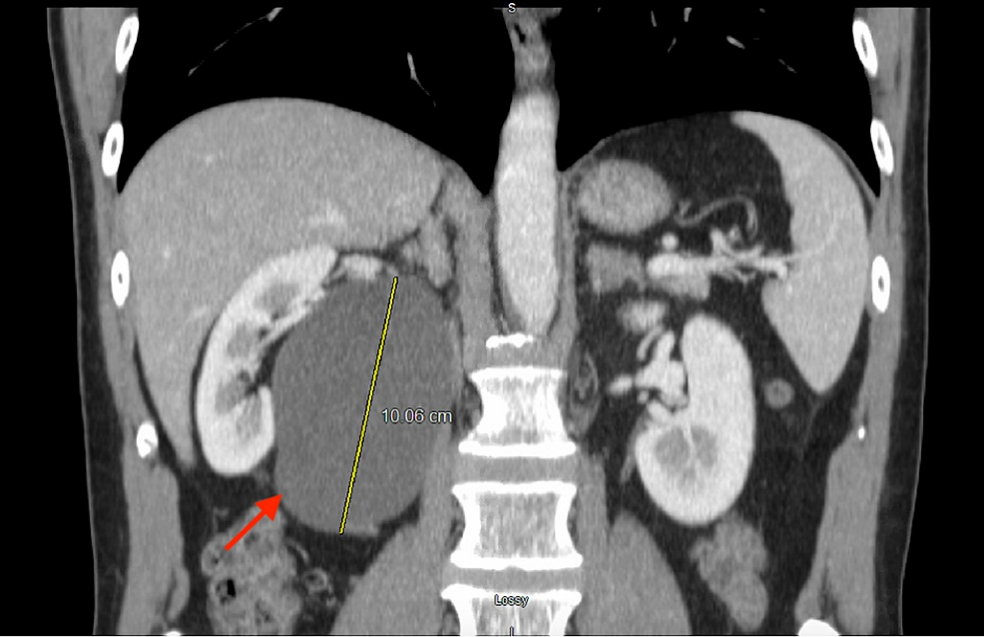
Coronal CT scan image showing a cystic mass (red arrow) adjacent to the right kidney measuring approximately 10.1 cm in craniocaudal dimension. CT: computed tomography
